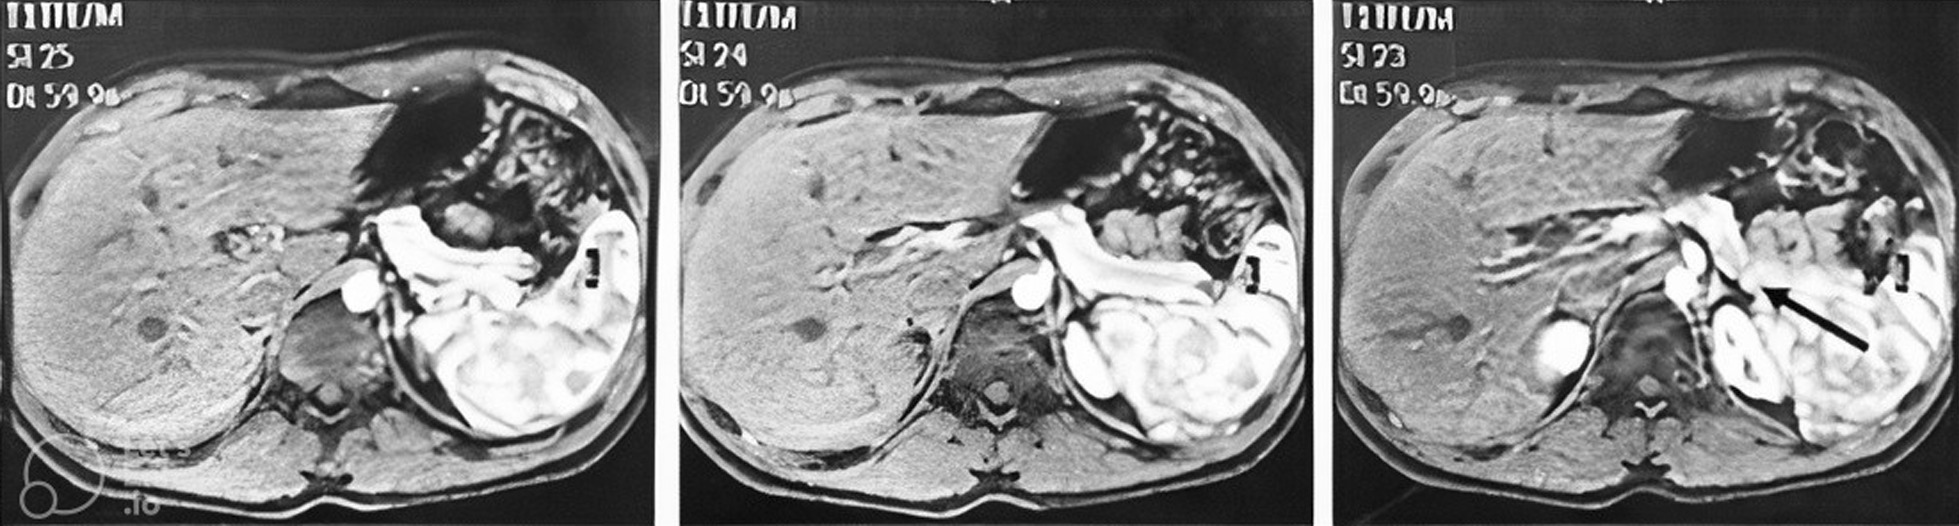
Magnetic resonance imaging of the patient’s pancreas. Arrow indicates the probable nodule in the body–tail transition
